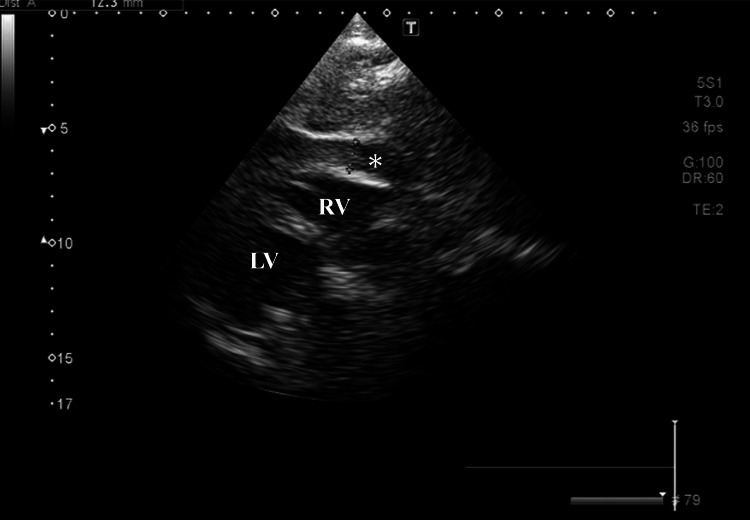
Transthoracic echocardiogram after the contrast-enhanced computed tomography (CECT) shows 13-mm pericardial effusion (asterisk). LV; left ventricle, RV; right ventricle.
